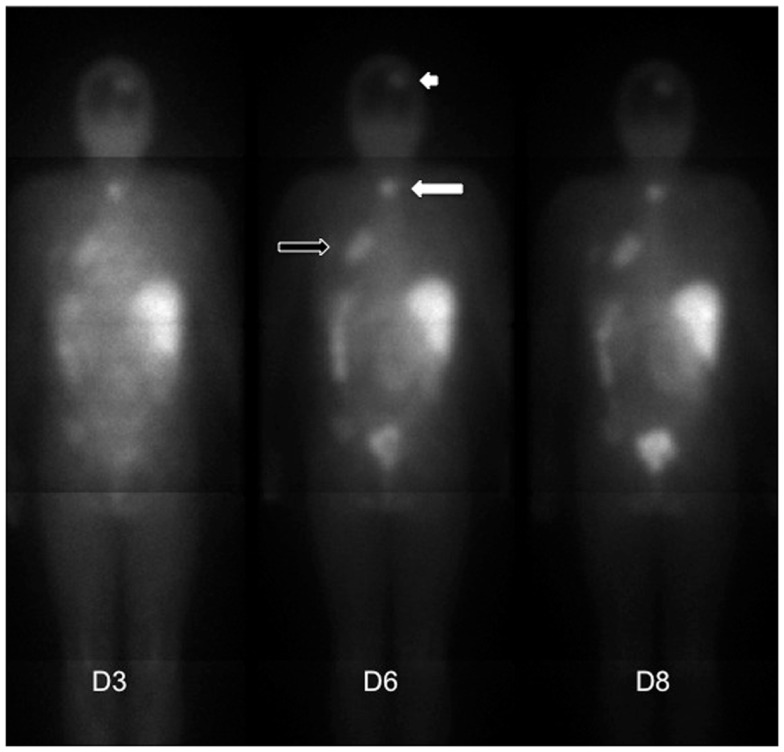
Immunoscintigraphies (posterior view) recorded in a patient with a metastatic CEA-positive small cells lung cancer. This patient was injected with the anti-CEA hMN14x734 bispecific antibody and a therapeutic activity of ^131^I-di-DTPA-indium. The immunoscintigraphies recorded 3, 6, and 8 days after hapten injection showed a good uptake in lung (black arrow), mediastinum (white arrow), and brain (white arrowhead) metastases.
